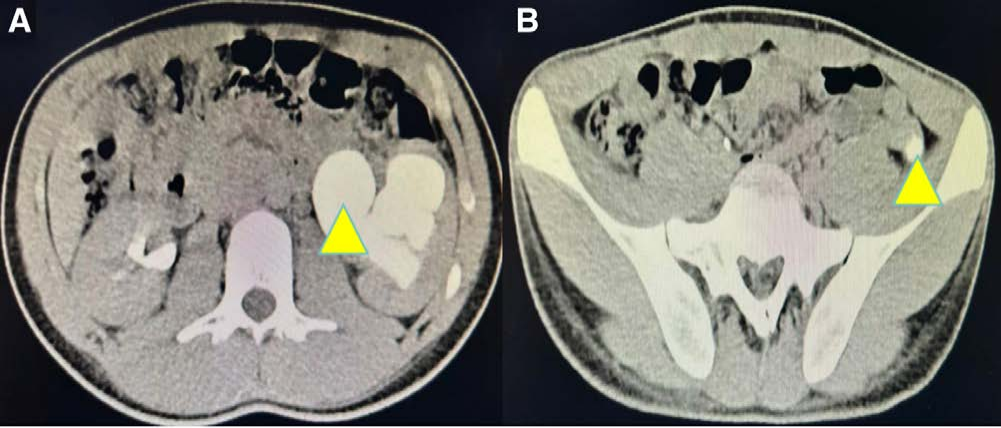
Computed tomography urography. (A) Severe left kidney hydronephrosis (indicated by yellow triangle); (B) Abnormal left ureteral shape and thickening of ureteral wall(indicated by yellow triangle).
